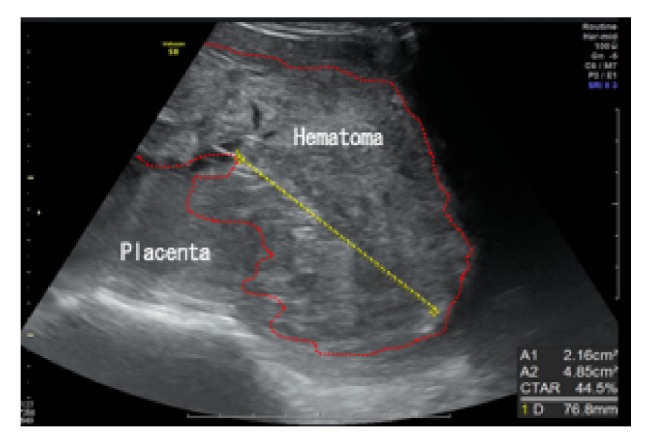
Ultrasound examination revealed a large placental mass with mixed high and low echogenicity measuring approximately 7.6 cm in thickness. The range of the presumed hematoma is indicated by the dotted lines.
